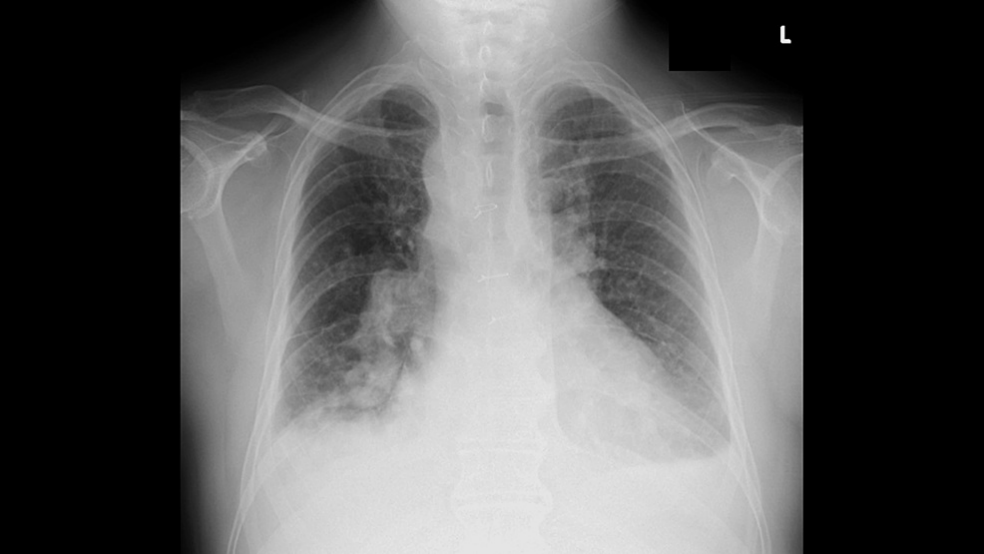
Chest X-ray on admission Cardiomegaly and bilateral pleural effusion are observed.
